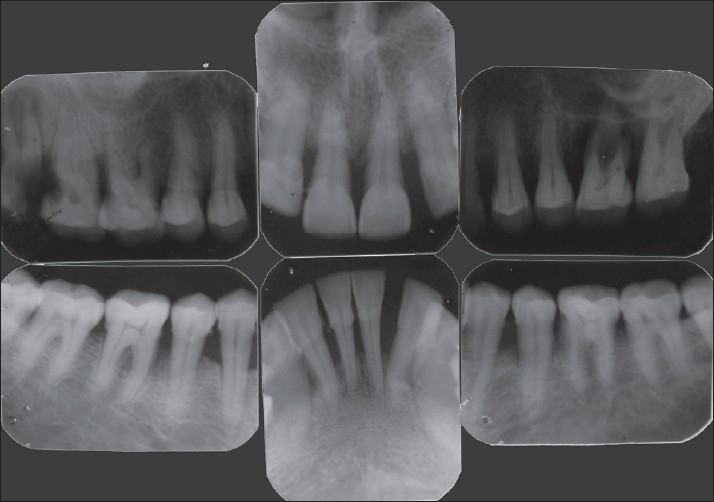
Fullmouth intraoral periapical radiographs
